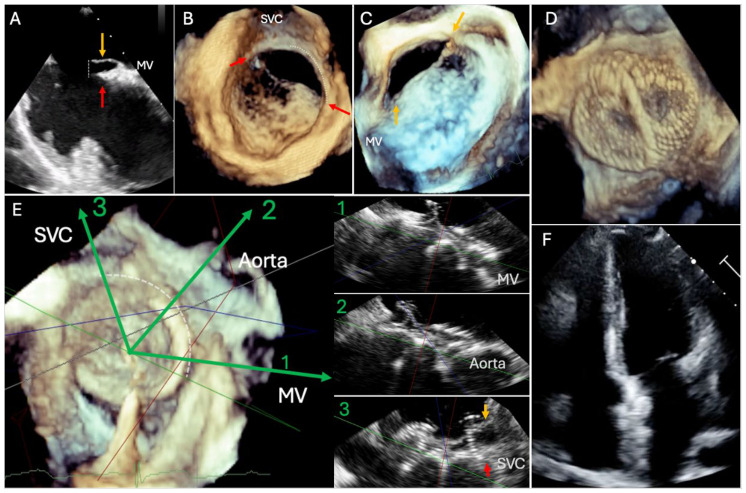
Malaligned septum. Image (A) depicts 2D 0-degree view of the atrial septum; septum primum (yellow arrow) is separated from the plane of the septum secundum (red arrow). The tissue separation measures 7 mm. In image (B), RA en face view, and image (C), LA en face view, the white dotted line depicts the off-set septum secundum rim from the RA side (between red arrows), and the septum primum tissue attachment on the LA side (yellow arrows). The defect was balloon sized (stop flow technique) and a device size chosen as balloon waist diameter +4 mm. Image (D), device deployed LA view. Image (E), careful step-by-step interrogation using multiplanar reconstruction of the 3D image to ensure that both primum and secundum tissue have been captured between the device discs; sweep from MV to SVC 1–3 (image 3 shows primum yellow arrow and secundum, red arrow, with LA and RA device discs positioned correctly). Image (F), follow up transthoracic echo at six months depicts good device position and reduction in right heart size. SVC—superior vena cava; MV—mitral valve.
